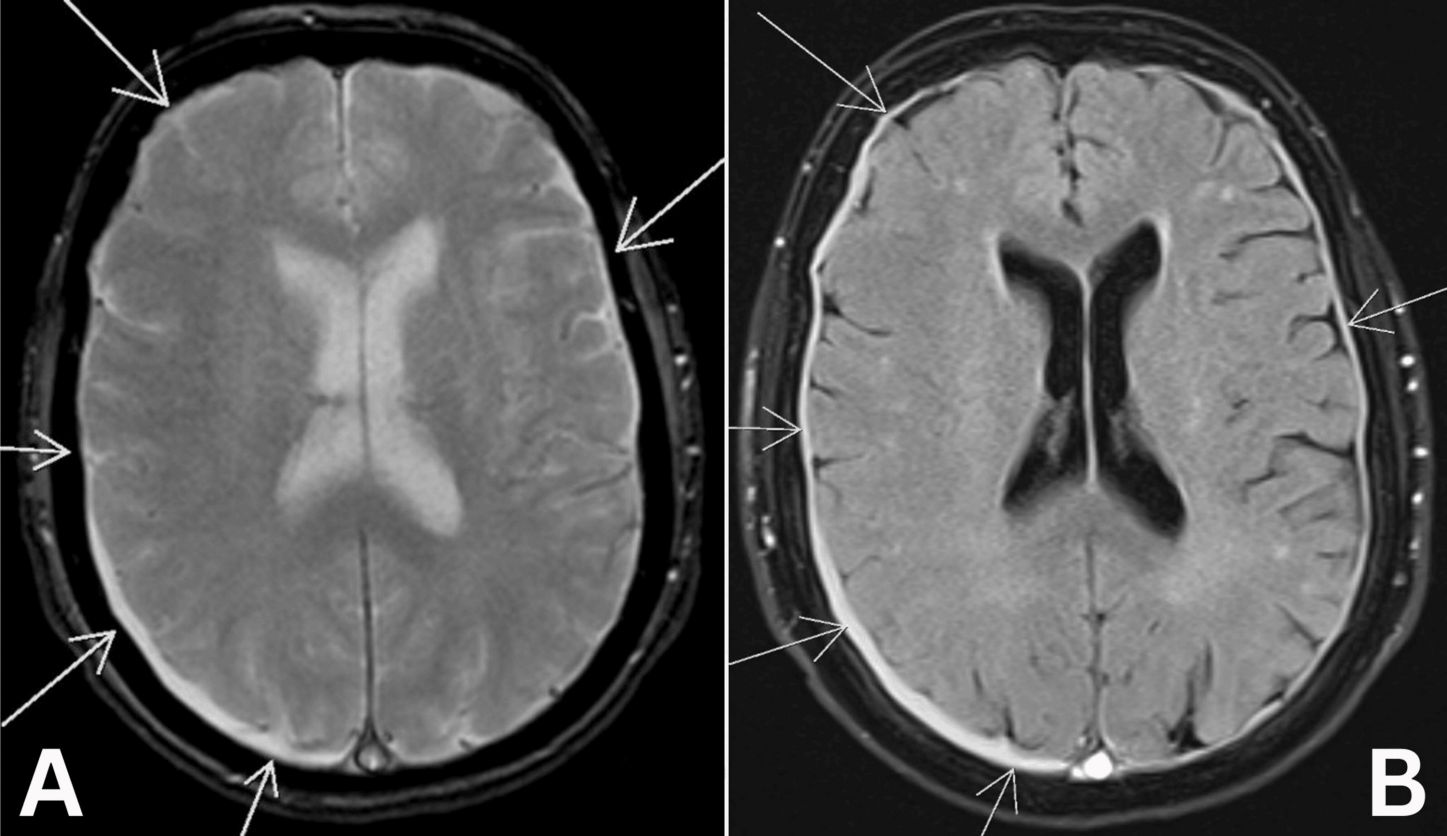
Axial MRI head images demonstrating bilateral subdural hygromas. (A) T2*-weighted gradient echo sequence showing bilateral subdural collections (arrows), more prominent on the right. (B) Fluid-attenuated inversion recovery (FLAIR) sequence showing the same subdural collections (arrows).
